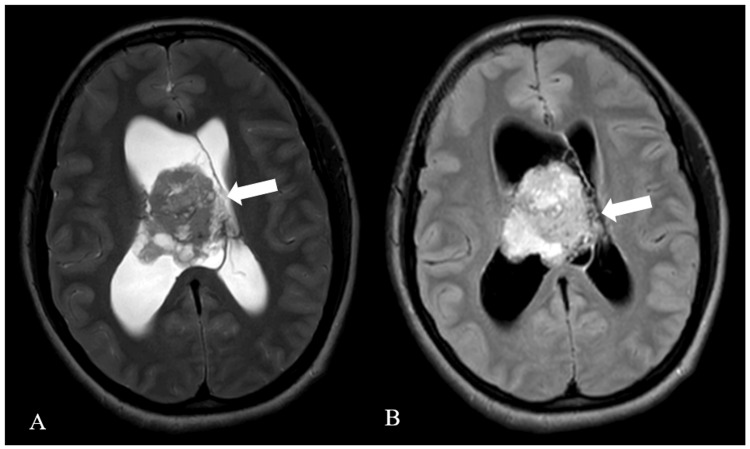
T2-weighted MRI. T2WI axial (A) and FLAIR axial (B) MRI: Magnetic resonance imaging; T2WI: T2-weighted image; FLAIR: Fluid-attenuated inversion recovery
